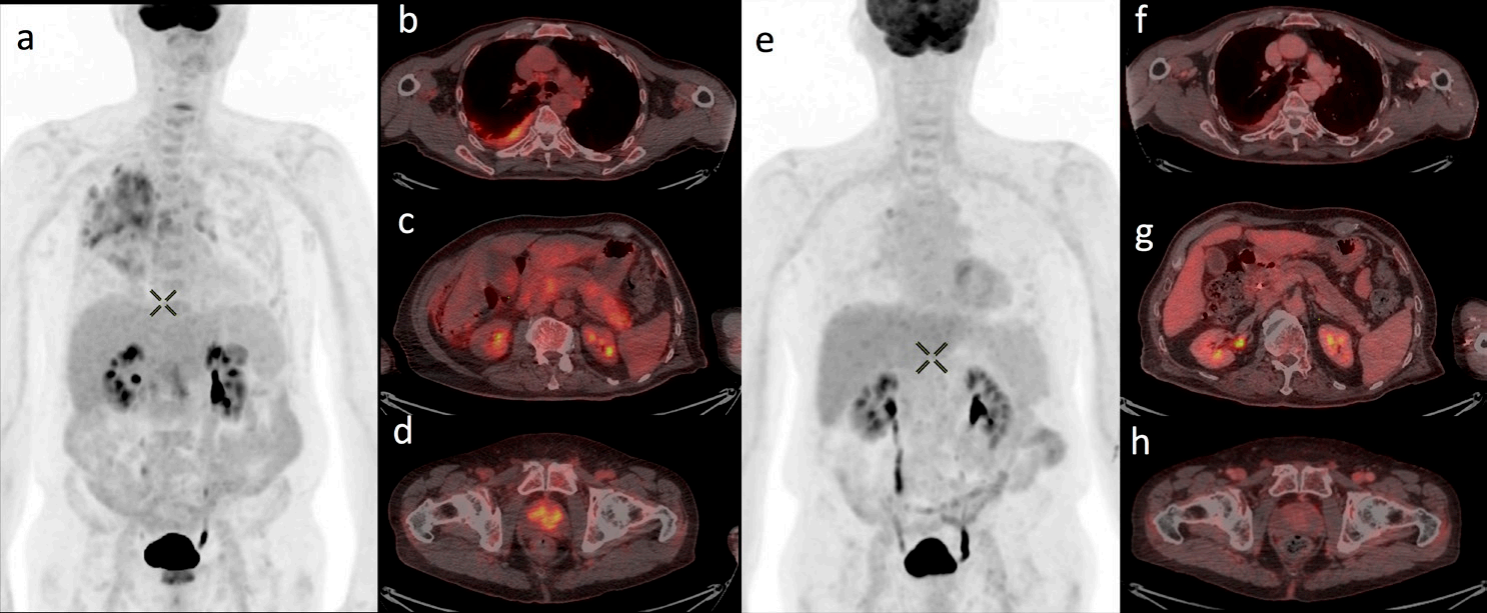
FDG PET-CT maximum intensity projection images before (a) and 10 weeks following initiation of steroid therapy (e). On the pre-treatment scan, there is avid FDG uptake within the right pleura (b), head and tail of the pancreas (c) and prostate gland (d). The post-treatment scan demonstrates decreased uptake in each of these areas (f, g, h)
